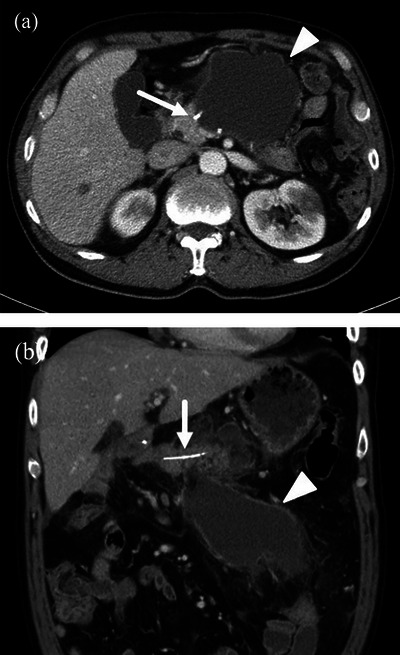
(a) Axial multidetector computed tomography view in the patient who underwent distal pancreatectom is shown. The arrow indicates the pancreatic stump by the linear stapler, and the arrowhead indicates the pancreatic fluid collection with an encapsulated wall. (b) Reconstructed coronal multidetector computed tomography view in the patient who underwent subtotal stomach‐preserving pancreatoduodenectomy is shown. The arrow indicates the internal stent in the pancreatojejunostomy, and the arrowhead indicates the pancreatic fluid collection with an encapsulated wall.
